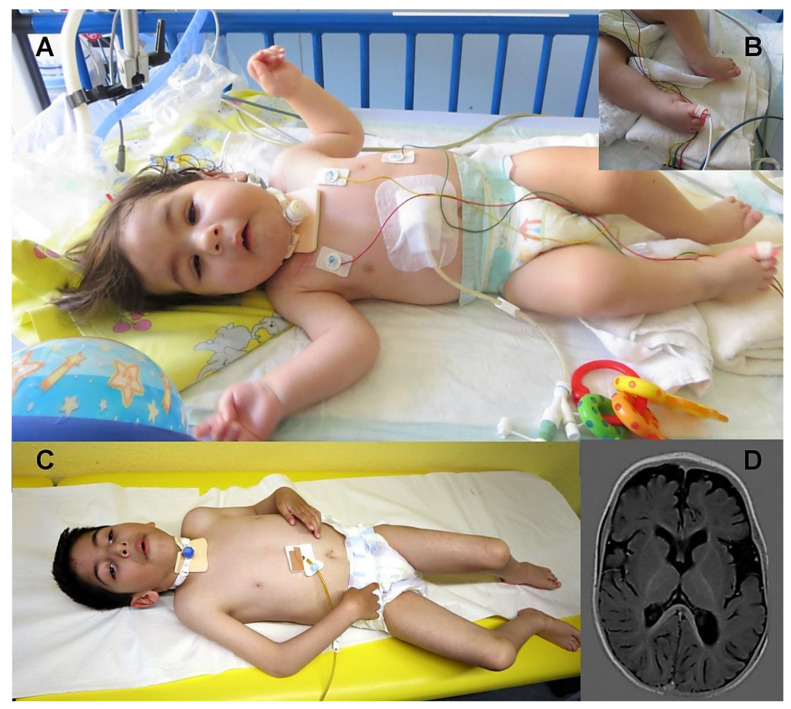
Clinical findings in the *SLC18A3* patient. (A) Patient at the age of 11 months displaying facial features including high arched palate (not to be seen), hypomimia, both-sided ptosis and club feet (B). Feeding difficulties lead to percutaneous endoscopic gastrostomy (PEG)-tube feeding, and a tracheostoma was placed due to respiratory crises with apnoea. (C) At the age of 5 years, the facial hypomimia and ptosis persisted. Moreover, the patient developed joint contractures in the lower extremities (knee joints) and still needed tracheostoma. (D) Magnetic resonance imaging (MRI) performed at the age of 11 months revealed volume reduction with bitemporal accent.
